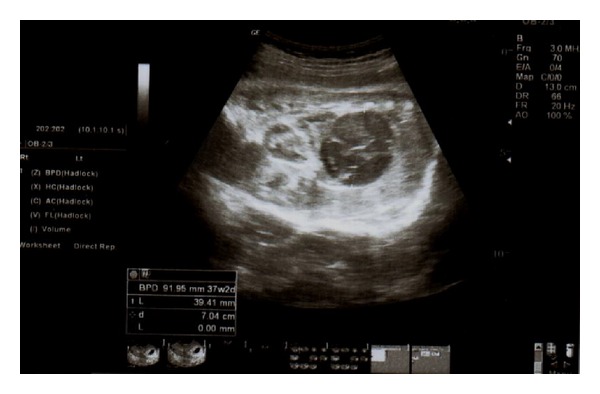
Ultrasonographic appearance of cystic neuroblastoma at 37 weeks of gestation.
